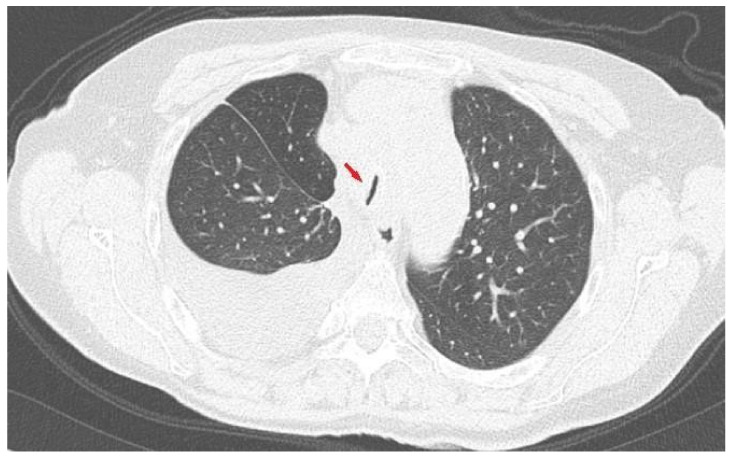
Chest computed tomography (CT) scan: axial lung image showing huge reduction (arrow) of the trachea lumen (2 mm) due to mediastinal pathological tissue.
